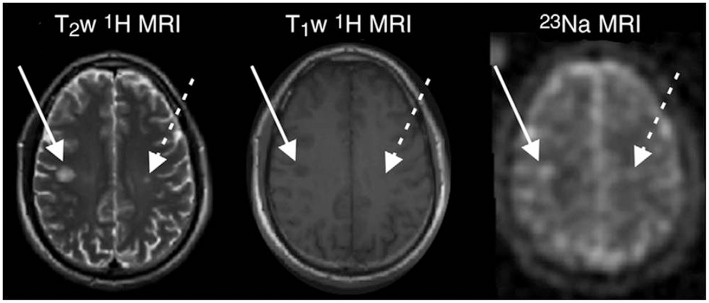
MR images in a 33-years-old man with early RRMS. Examples of substantial sodium accumulation in two macroscopic T2 lesions with two different signal intensity patterns at T1-weighted imaging: one lesion was hypointense (solid arrows) and one was isointense (dashed arrows) to normal-appearing white matter on T1-weighted image. Figure reproduced from (99) with permission of the Radiological Society of North America (RSNA).
